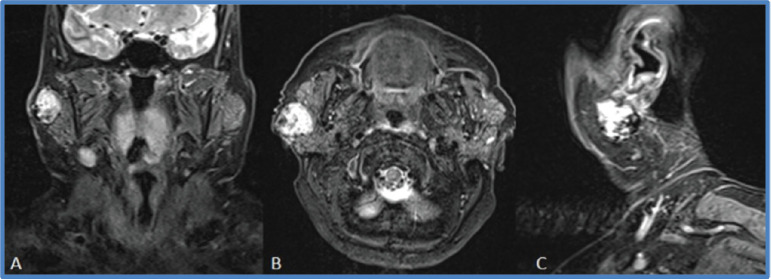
MRI sections. (a): coronal, (b): axial and (c) sagittal in STIR sequence. Right superficial parotid nodule, with well-defined edges, a predominantly hyperintense, heterogeneous signal and papillary-like projections inside.
